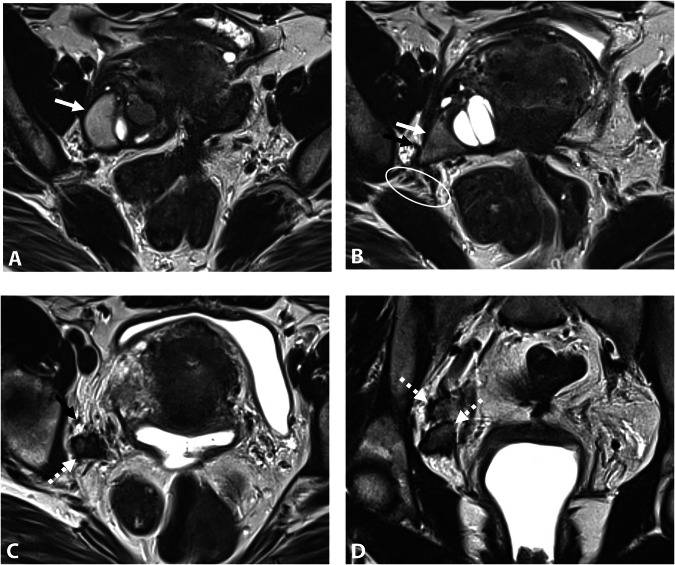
DE in a 29-year-old woman with deep dyspareunia, right sciatica, pain in the right inner thigh, and difficulty in walking. A–C Axial and (D) coronal T2W MR images show subperitoneal endometriosis lesion along and from the right adnexa (A, B, white arrows), extending to the right distal mediolateral parametrium (C, D, dashed arrows) and to the right obturator nerve (B, C, black arrows). The lesion is in contact with the right sciatic nerve posteriorly (B, circle), with an extrinsic traction effect but no involvement. After the failure of the medical treatment, the patient underwent surgery, including hysterectomy, right adnexectomy, right parametrectomy, ureterolysis, and dissection of the obturator nerve, which was entrapped by the mass
